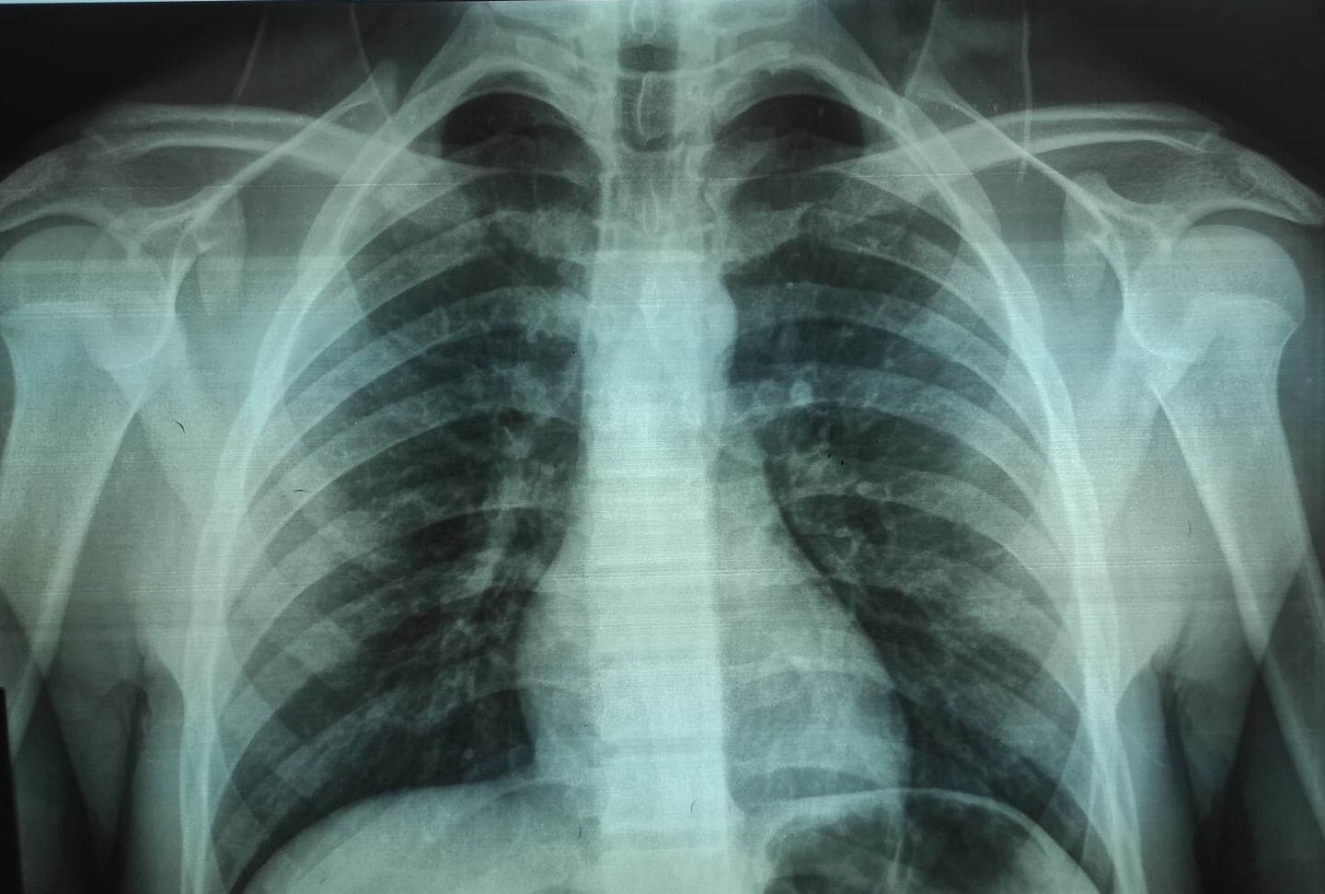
A chest radiograph of a 17-year-old boy showing no detectable abnormalities.
